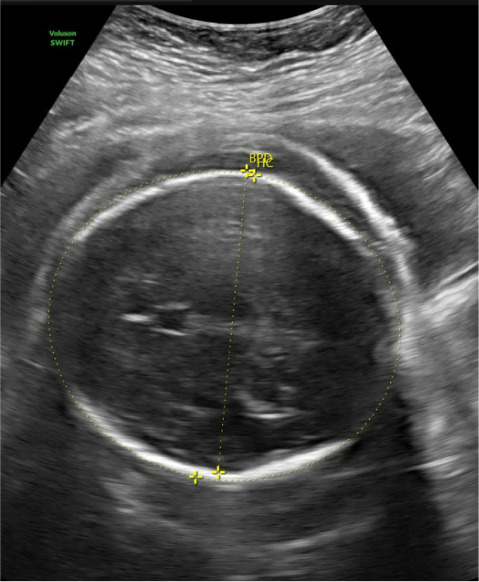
Ultrasound demonstrating scalp edema.
